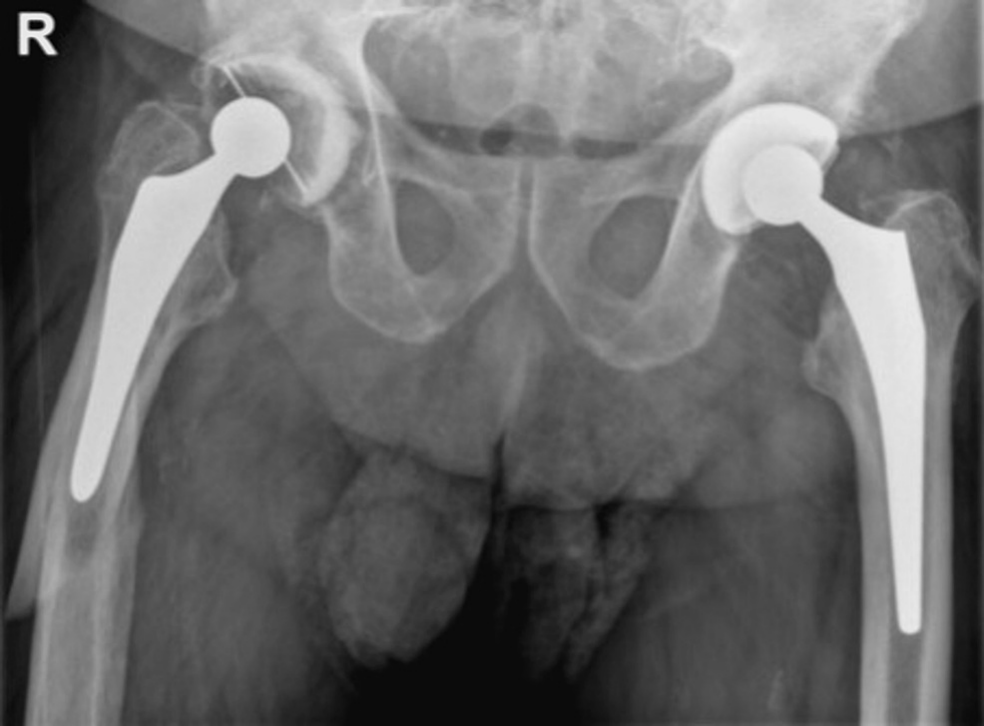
Case B, X-pelvis pre-OR X-ray showing a periprosthetic femoral fracture of the right femur, Vancouver B1 type of fracture
